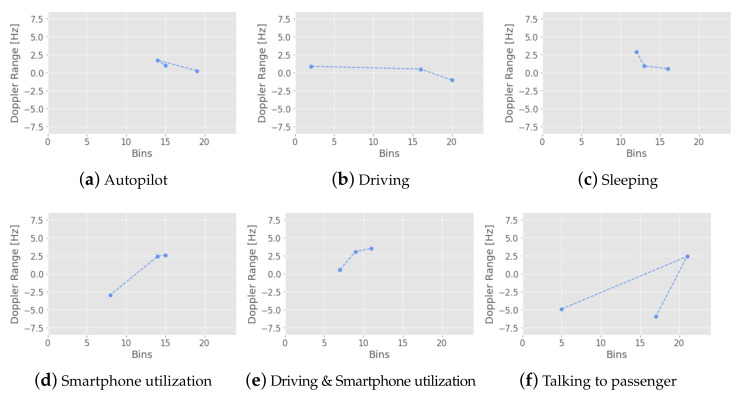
Range-Doppler trajectories of six (a–f) in-cabin activities calculated using the method of [32]. Each trajectory contains a single frame (0.34 s).
